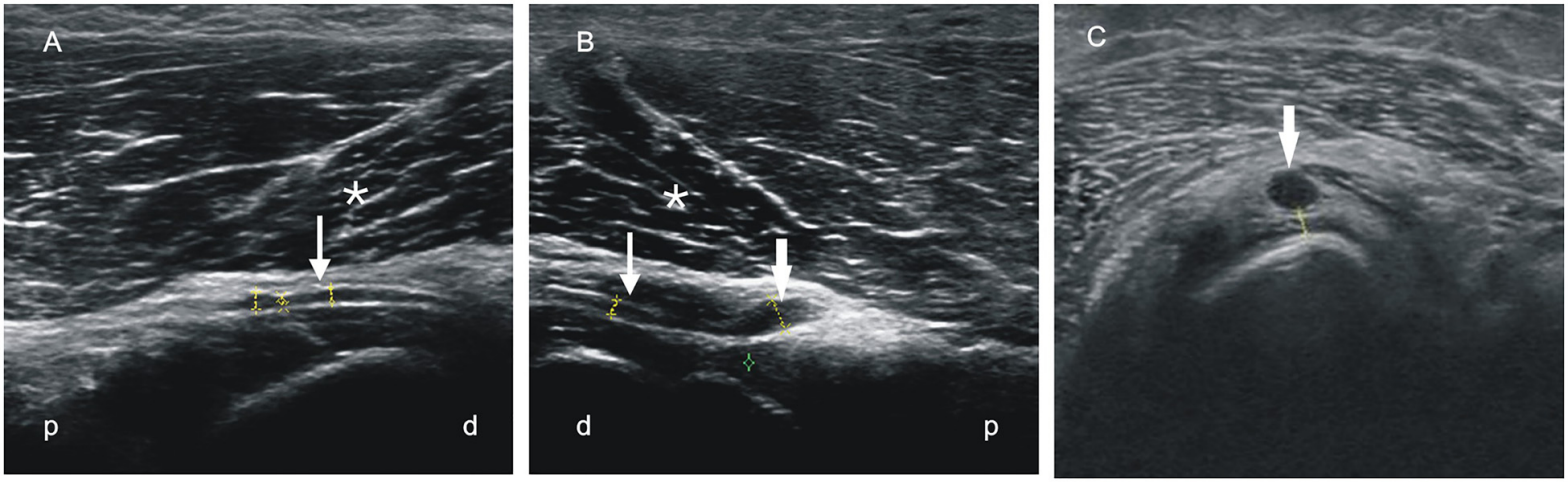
Sonographic studies of the patient with a posterior interosseous nerve (PIN) syndrome. (A, B) Longitudinal study of healthy PIN (A) and the affected side (B) with significant swelling (thick arrow) before entering the supinator muscle (asterisk). (C) Transversal study with a significant swelling of the PIN (arrow) anterior to the supinator muscle. p—proximal, d—distal
